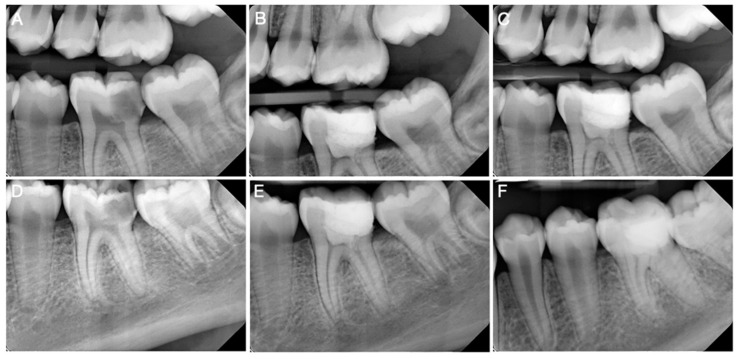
Clinical case using dHACMs for vital pulp therapy. (A,D) Preoperative radiograph showing the mandibular left first molar with extensive caries involving the distal pulp horn. The tooth was diagnosed with symptomatic irreversible pulpitis and symptomatic apical periodontitis. (B,E) Postoperative radiograph after the removal of both caries and inflamed coronal pulp tissue, the placement of dHACMs, and restoration. (C,F) Three-month follow-up showing no periapical lesion. The tooth was negative to percussion and palpation and positive to electric pulp testing.
